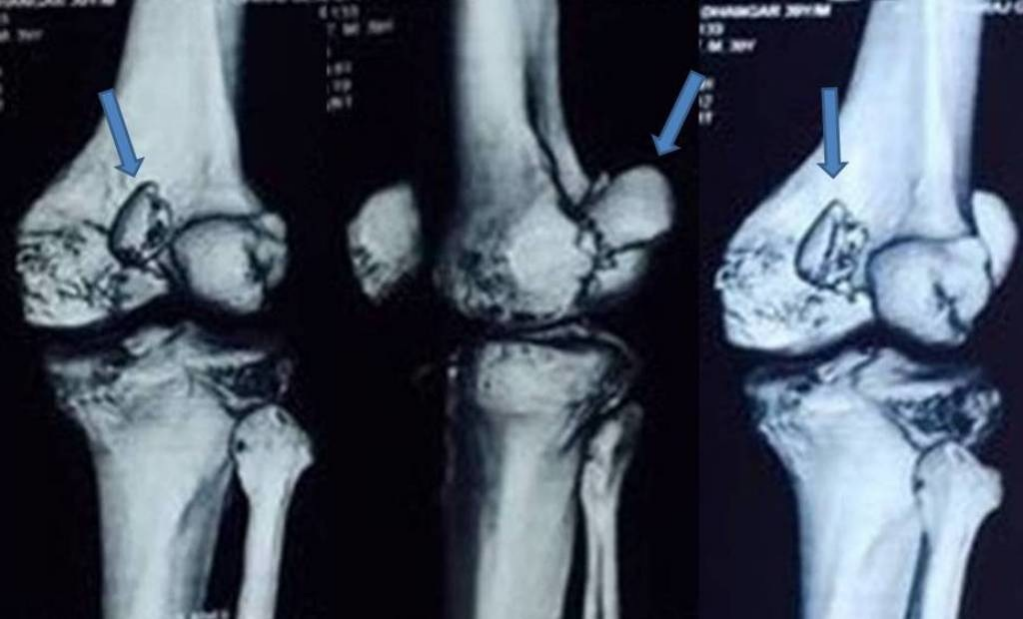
A three-dimensional computed tomography (CT) scan image showing the distal femur medial condyle Hoffa type II(C) fracture
